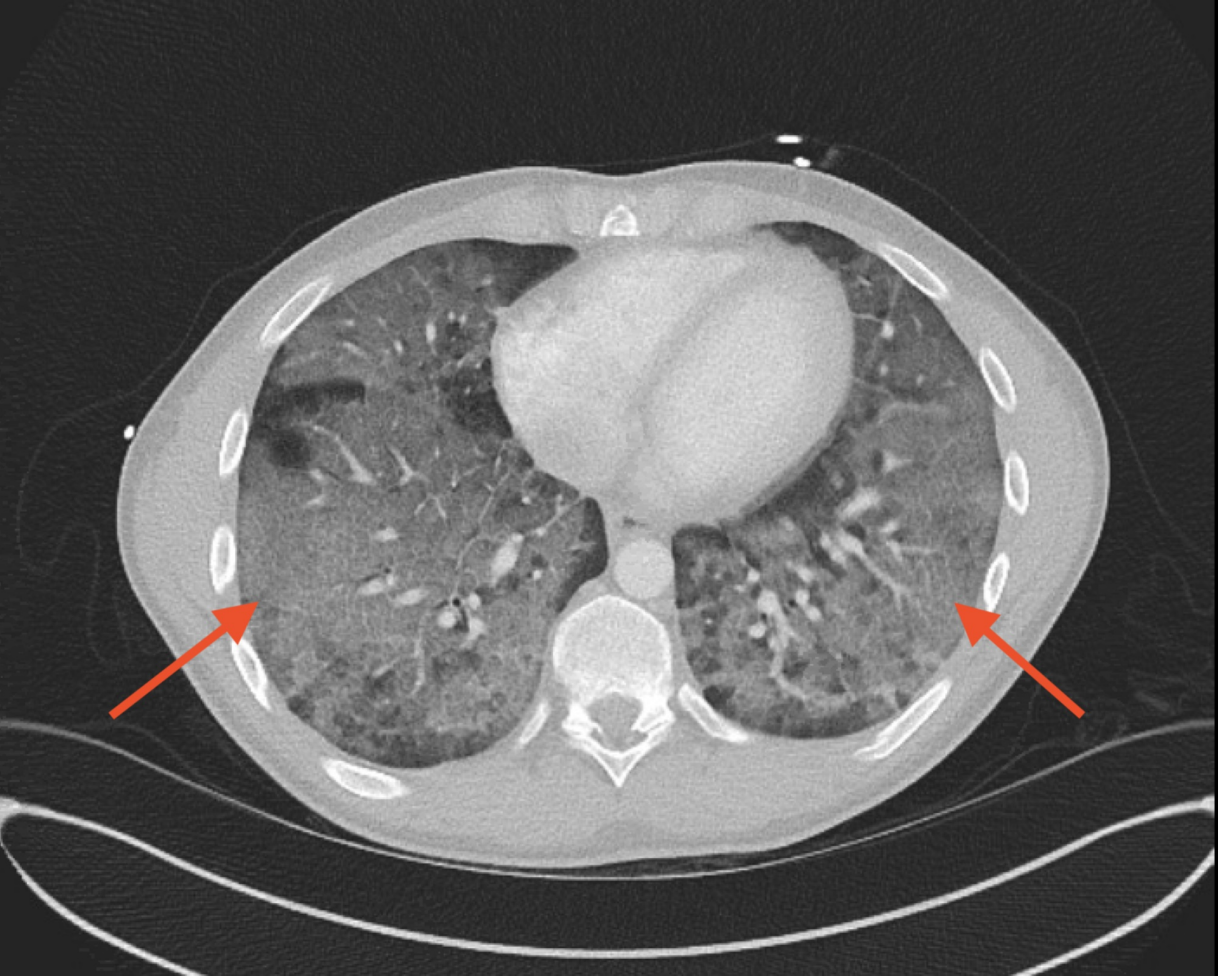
Computed tomography (CT) of the chest on Day 2 of admission with rapidly worsening ground-glass opacities throughout the lungs (red arrows).
